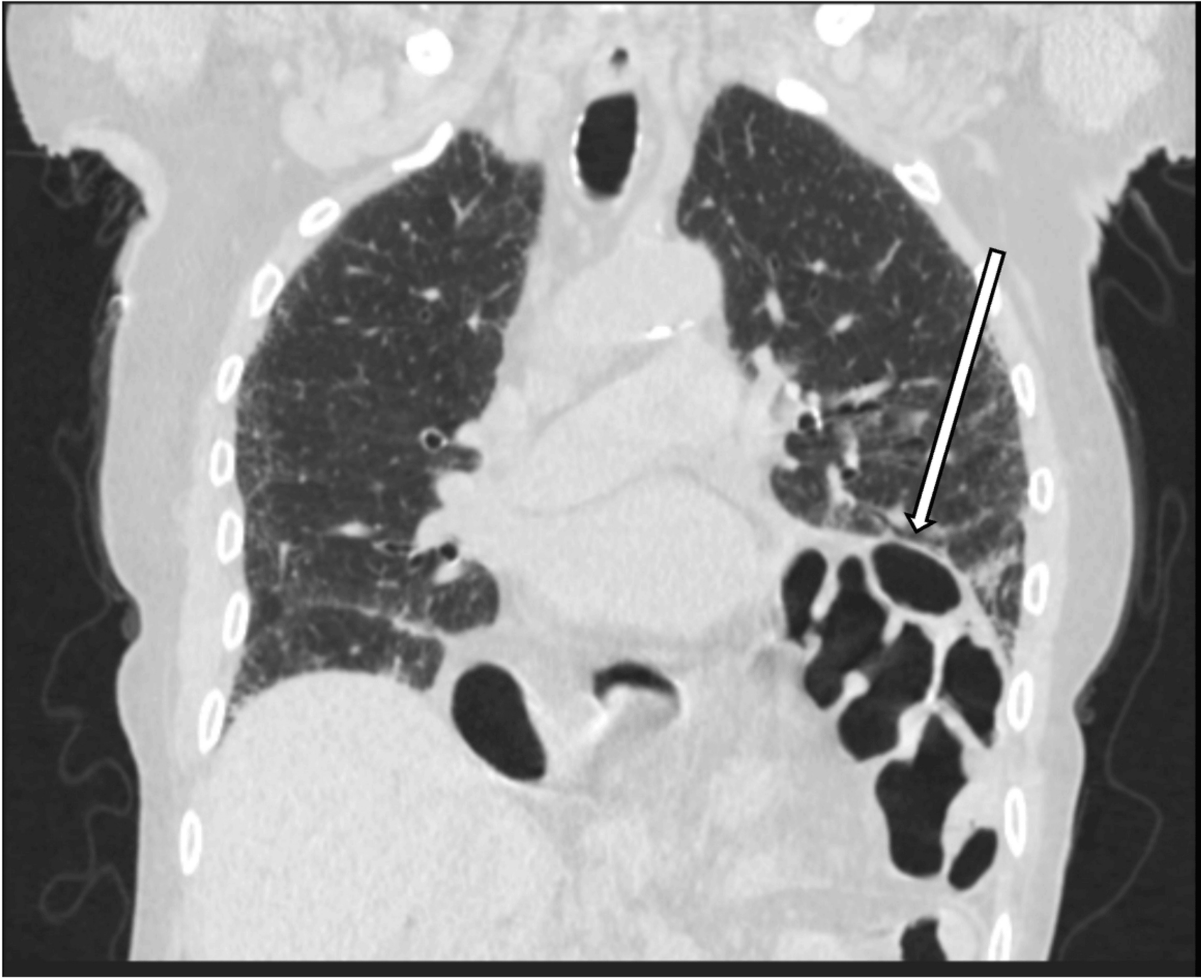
CT Chest Axial view in the lung window showcasing herniation of bowel contents into the left hemithorax.
